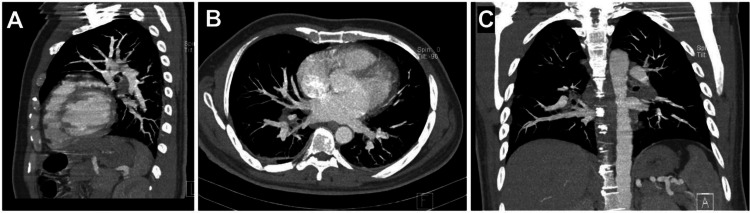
CT angiography of the lungs: (A) Sagittal view, (B) Axial view, (C) Coronal view. No evidence of pulmonary thromboembolism (PTE) or any other complications, including fat embolism syndrome (FES), was observed.
